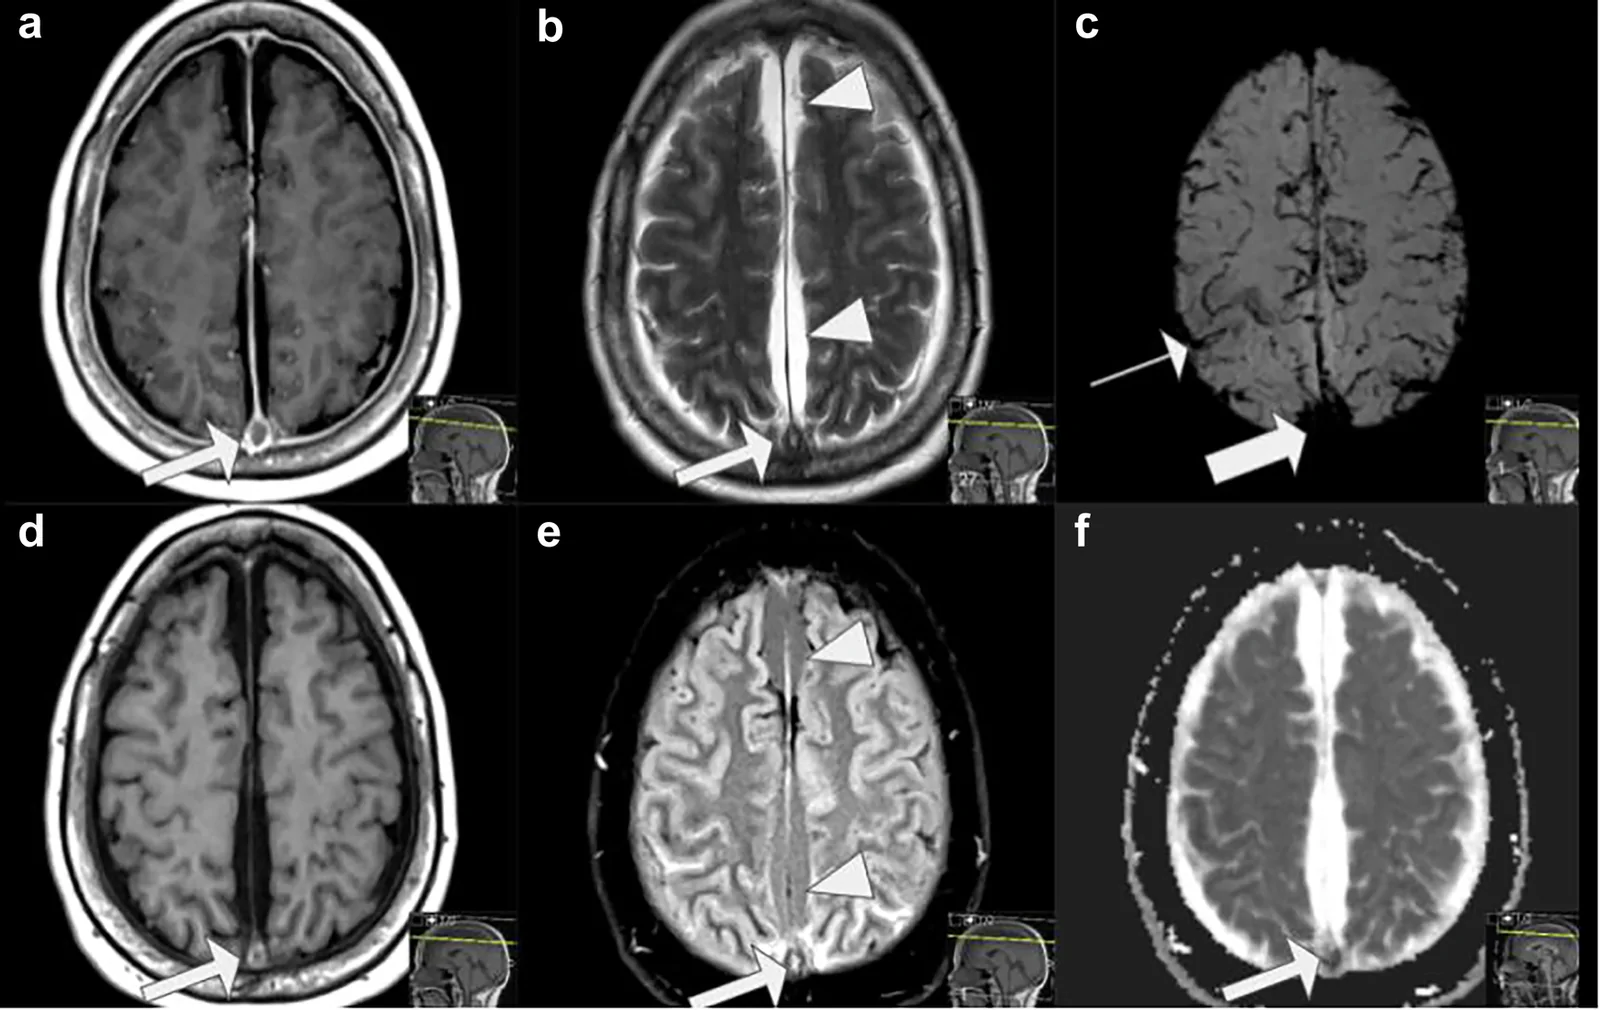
SSS thrombosis with subdural hygromas indicating intracranial hypotension. Multisequence MRI demonstrates: a Post-contrast T1 shows a filling defect with an empty delta sign (arrow). b T2-weighted image reveals heterogeneous signal in thrombus (arrow) and bilateral subdural hygromas with slightly elevated signal (arrowheads), not indicating frank hemorrhage. c SWI demonstrates dilated, stagnant cortical veins (thin arrows) and hypointense thrombus (thick arrow). d Non-enhanced T1 shows a heterogeneous signal within the thrombus (arrow). e T2-FLAIR demonstrates mixed signal intensity thrombus (arrow) and subdural hygromas with slight hyperintensity (arrowheads). f ADC map shows restricted diffusion within the SSS thrombus (arrow). The presence of subdural hygromas with venous stasis suggests underlying intracranial hypotension as a predisposing factor for thrombosis
